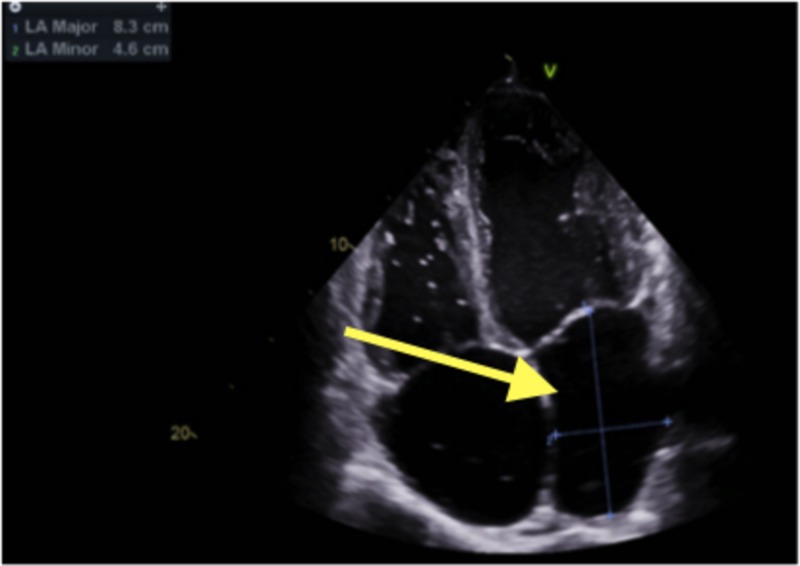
Echocardiogram: Arrow pointing toward dilated left atrium
